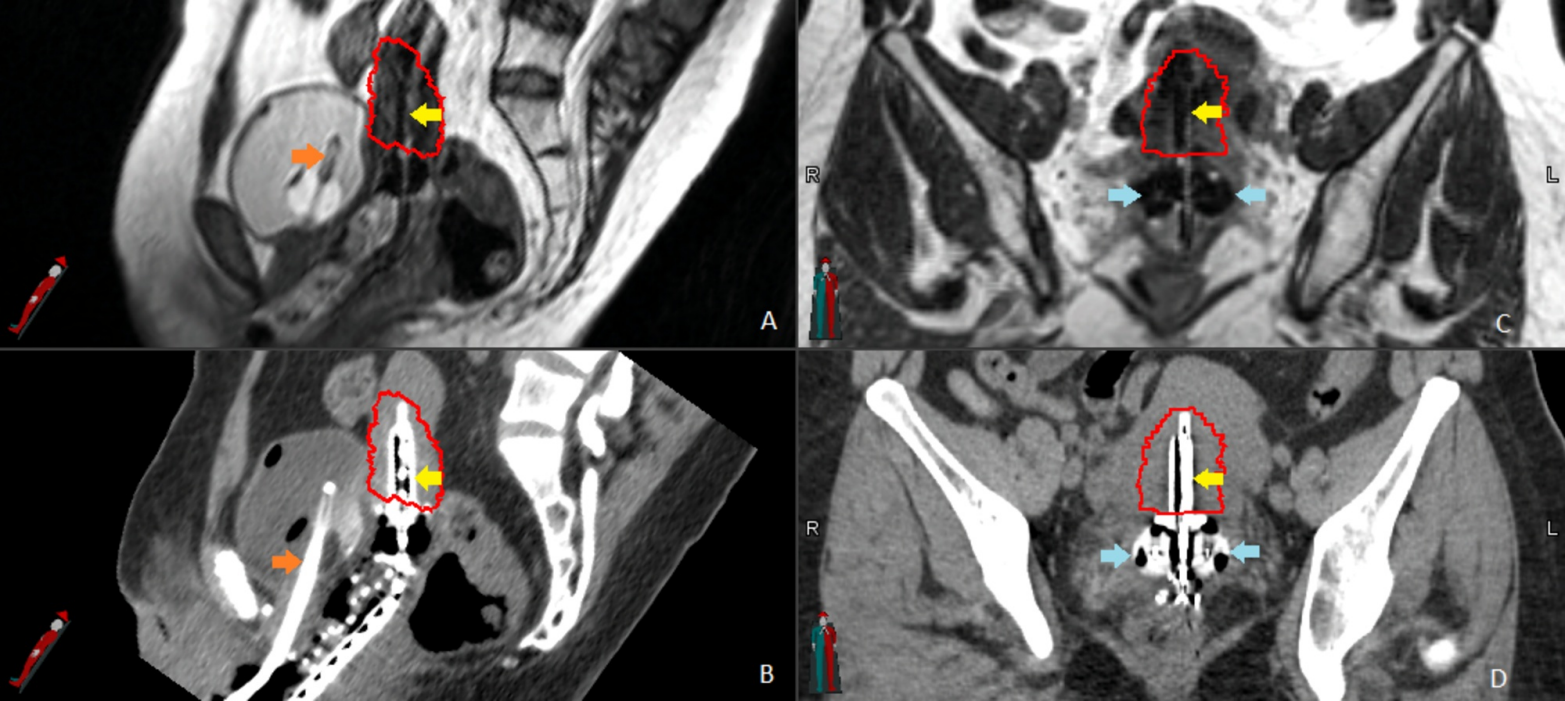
Magnetic resonance-guided brachytherapy. (A) and (B) show the uterine tandem (yellow arrows) and foley catheter (orange arrows) in the sagittal plan on the magnetic resonance imaging (MRI) and computed tomography (CT). The high-risk clinical target volume (HR-CTV) is outlined in red. (C) and (D) show HR-CTV, tandem (yellow arrows), and ovoids (blue arrows) in the coronal plane, again on the respective MRI and CT.
